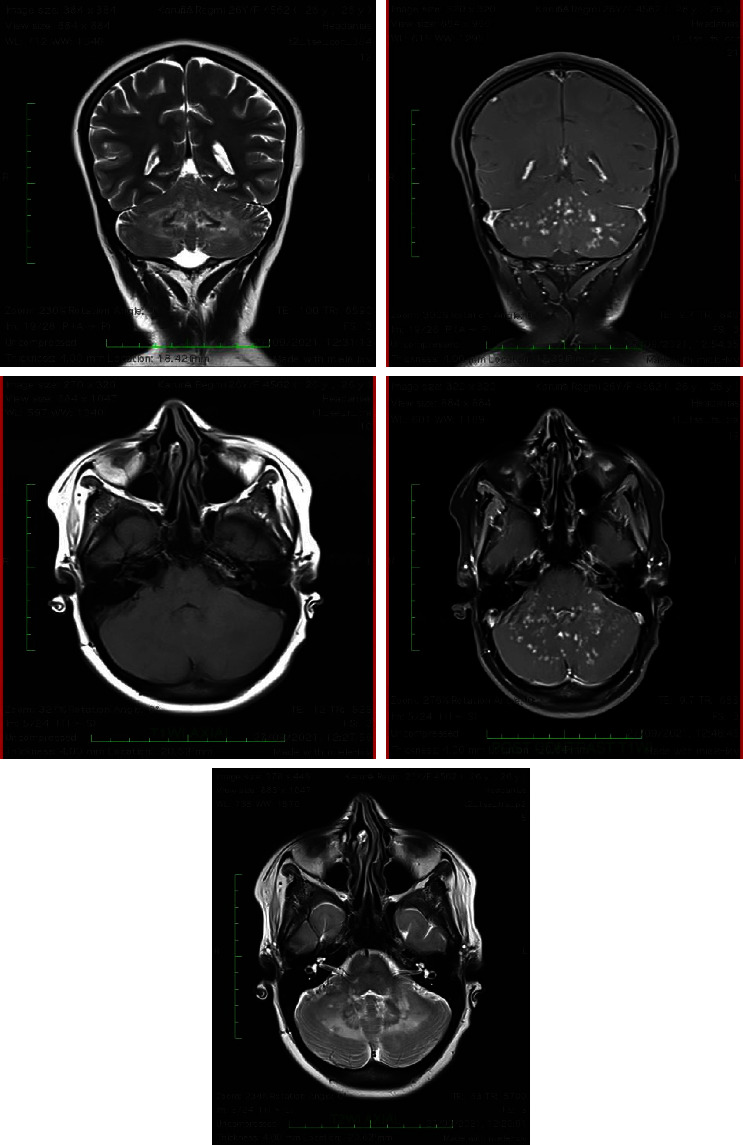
MRI brain images of the patient with CLIPPERS syndrome showing diffuse and patchy altered high T2/FLAIR signal intensity areas with multiple punctate, patchy, linear enhancement in bilateral middle cerebellar peduncles, adjacent bilateral cerebellum, vermis and pons, and left parietal lobe.
